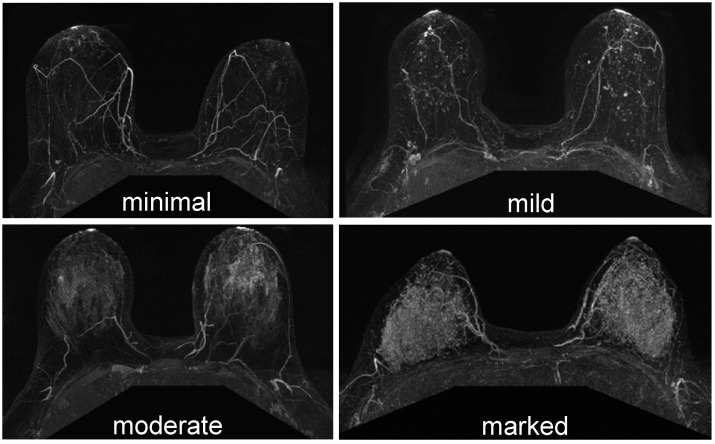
Examples from the Breast Imaging-Reporting and Data System (BI-RADS) lexicon for contrast-enhanced MRI, showing the different degrees of background parenchymal enhancement (BPE) in the breast: minimum, mild, moderate, and marked enhancement.
